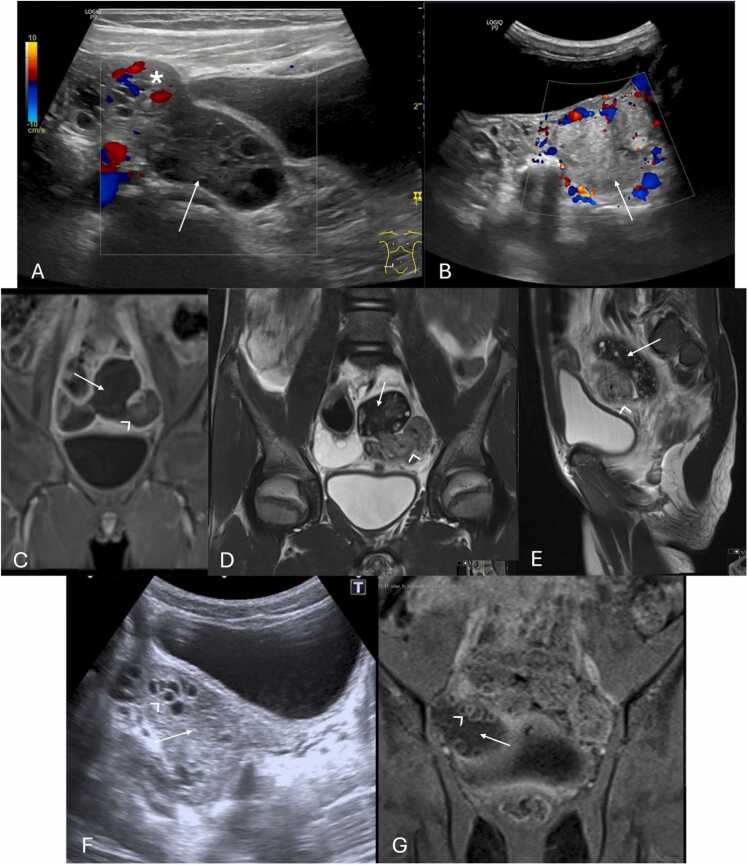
(A and B) Suprapubic pelvic ultrasound with color Doppler in different females, aged 14 and 8 years-old, respectively, depicting different examples of absent ovarian vascularity (arrows), presenting with loss of signal on Color Doppler, highly suggestive of ovarian torsion. In image (A) note the presence of flow within the vascular pedicle (*) of the adjacent fallopian tube, which on Spectral analysis (not shown here) presented with a high-resistive flow (Resistive Index > 0.7), indicating downstream obstruction of the blood flow. To confirm the clinical and radiological suspicion in patient (B), MRI was obtained pre-surgically. Coronal T1-weighted FS post-contrast (C) and T2-weighted (D and E) coronal and sagittal depict an enlarged left ovary (arrows) with loss of normal enhancement pattern after gadolinium injection as well as an abnormal T2 hypointensity. Please note the presence of a twisted ovarian pedicle, representing the whirlpool sign (arrowhead). (F and G) Same patient as in (B–E) 3 years after oophorectomy for surgically confirmed left ovarian torsion, now presenting with pain in the right iliac fossa. Transabdominal pelvic ultrasound and coronal T1-weighted FS post-contrast image depicting an enlarged right ovary (arrow), with heterogeneous parenchyma and peripherally displaced follicles, presenting with a hyperechoic rim, characteristic of the follicular ring sign (arrowhead). It was confirmed during surgery the presence of ovarian torsion.
